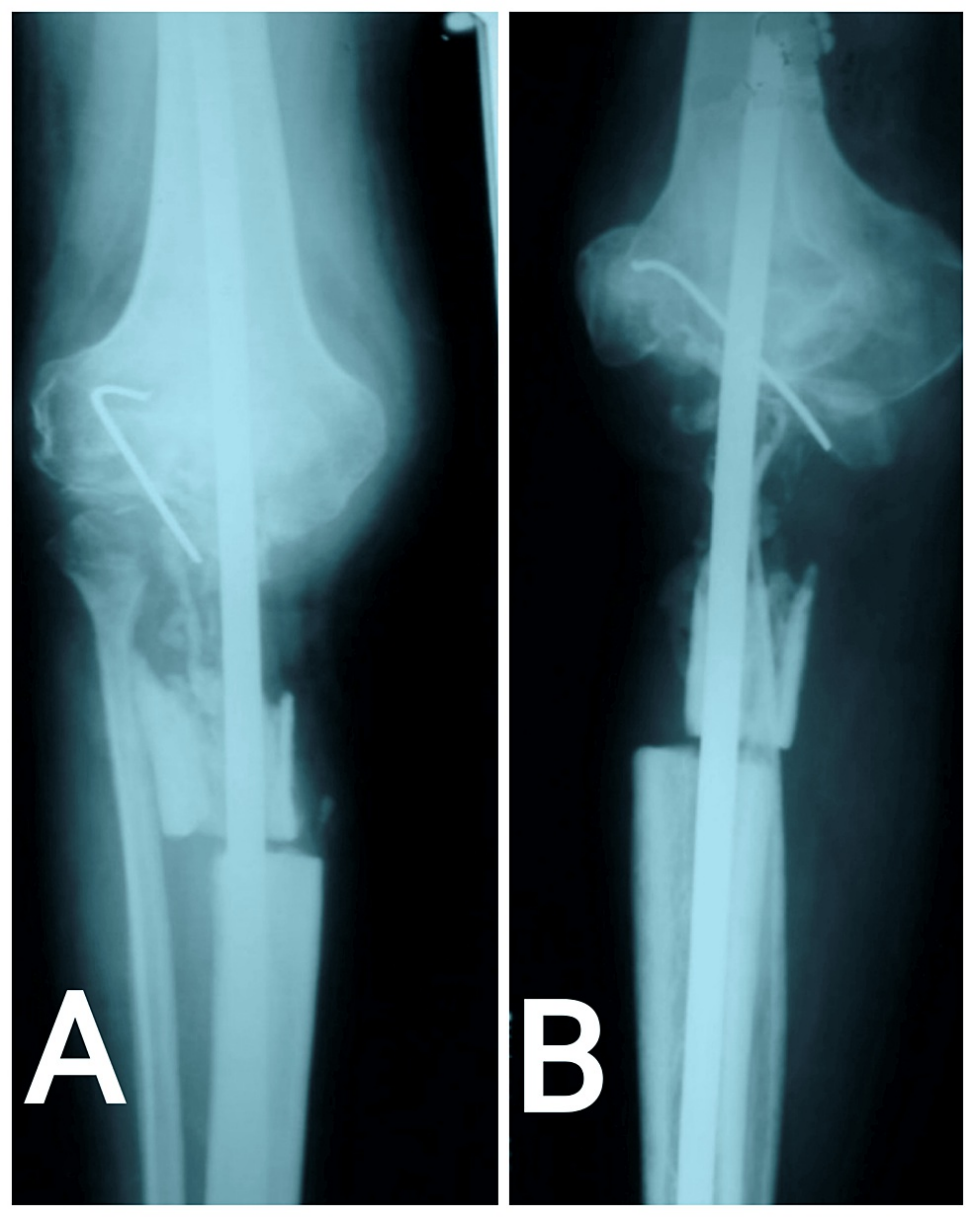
X-ray showing resection with bone grafting and arthrodesis with tibiofemoral nail. (A) Anteroposterior view; (B) Lateral view
